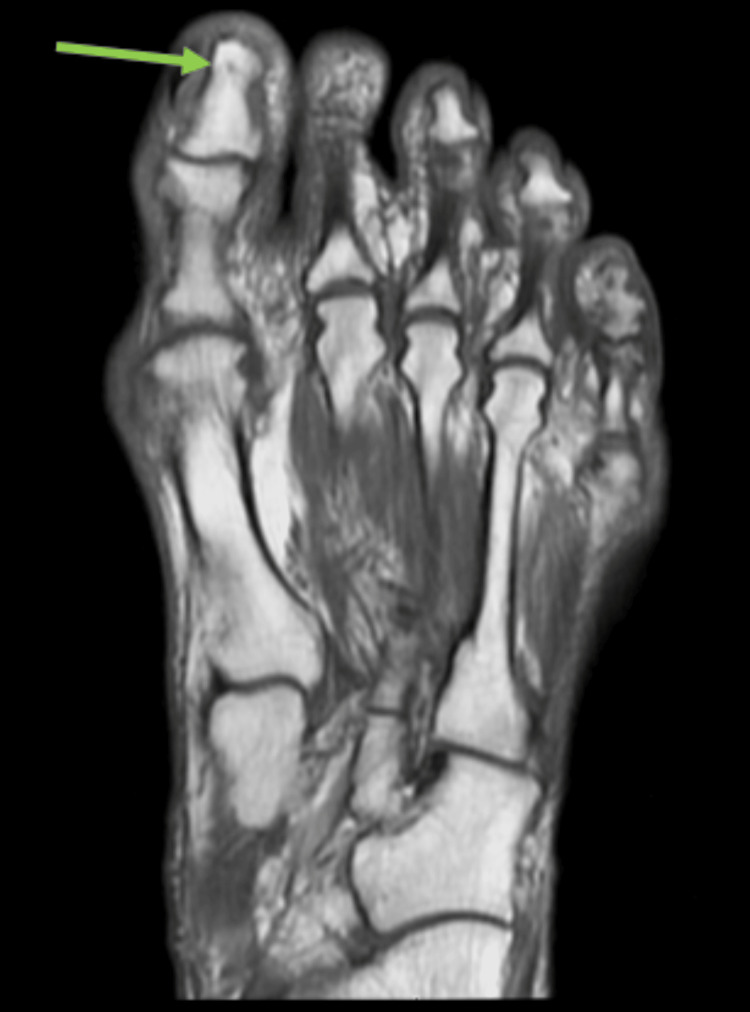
Magnetic resonance imaging of the left foot (with and without contrast) There is no evidence of osteomyelitis, abscess, fracture, or soft tissue infection (denoted by green arrow).
